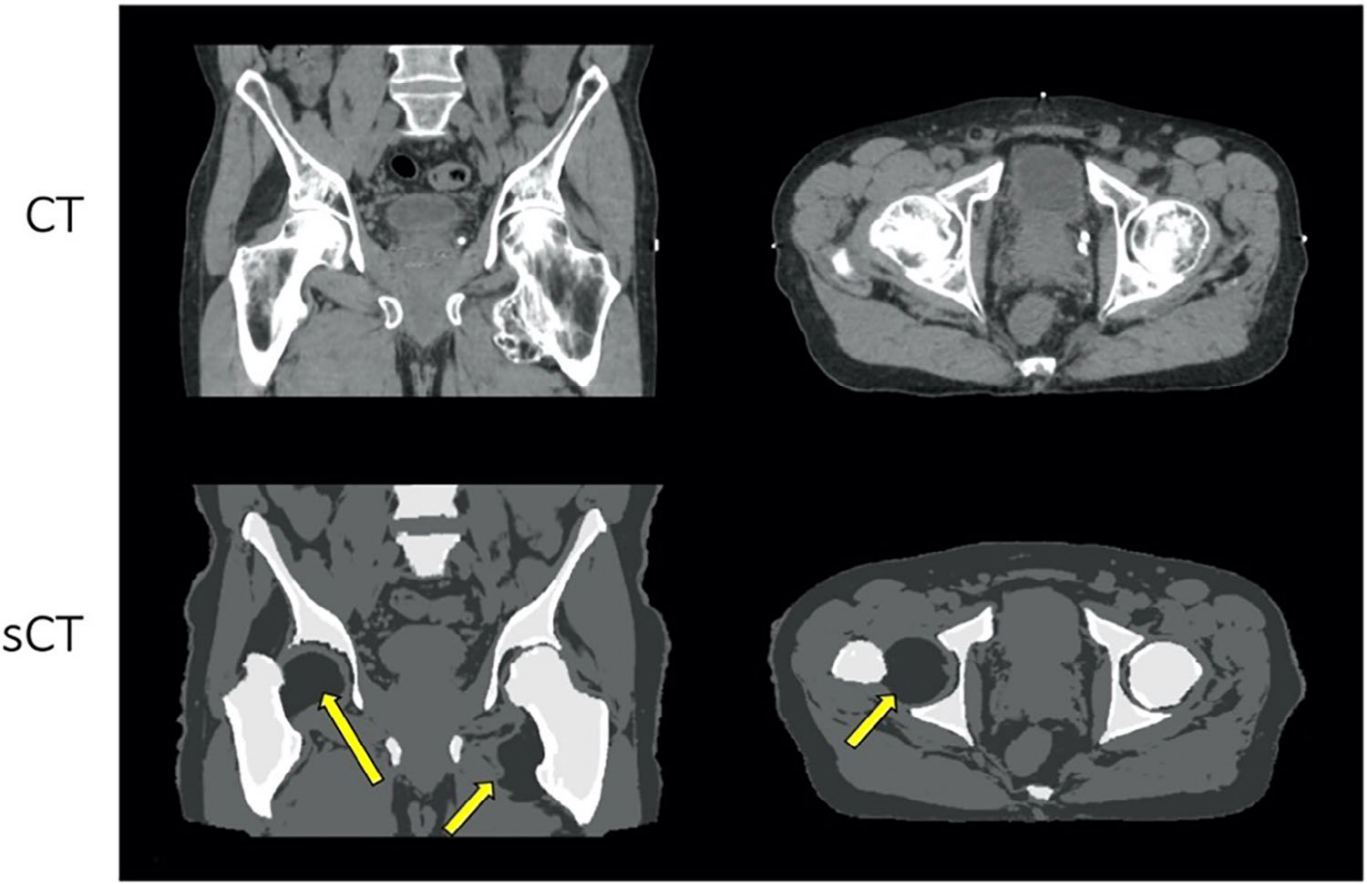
Example sagittal and axial slices of CT (top row) and sCT (bottom row) datasets for Patient #9 whose datasets exhibited the largest deviation in bone segmentation, which may have been caused by the abnormal shape of the left femoral bone which challenges atlas‐based reconstruction. CT, computed tomography; sCT, synthetic CT.
